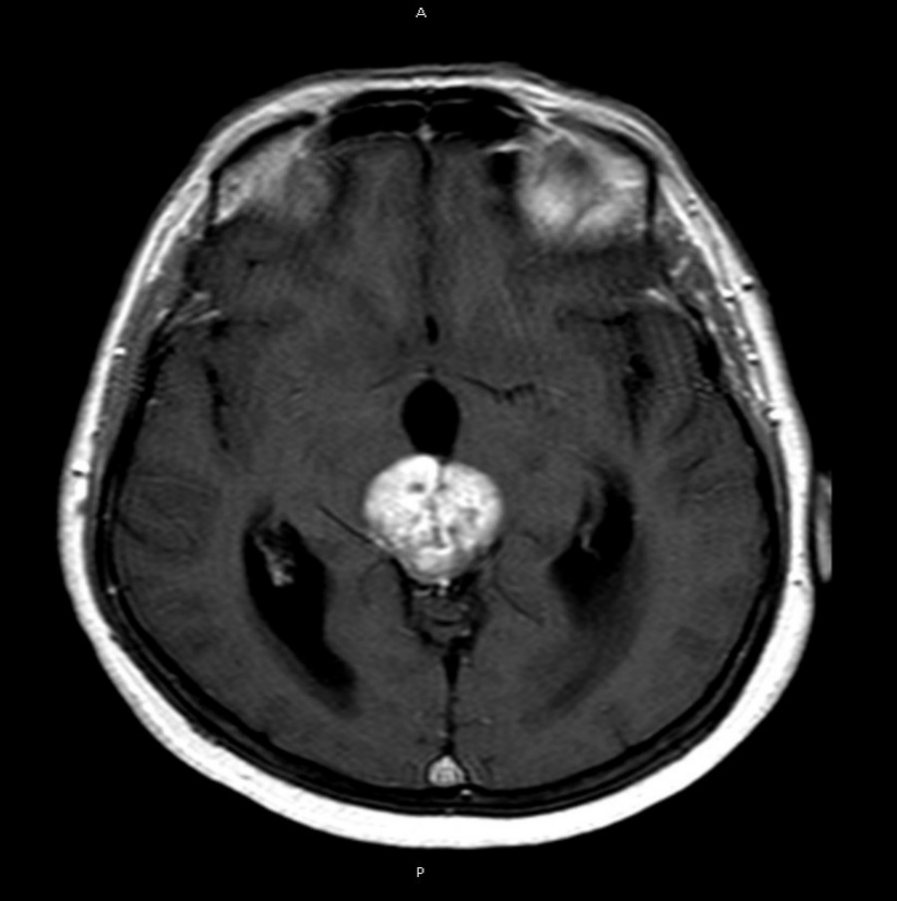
Preoperative MRI Preoperative axial MRI with contrast showing an enhancing pineal region lesion in the center of the picture.
